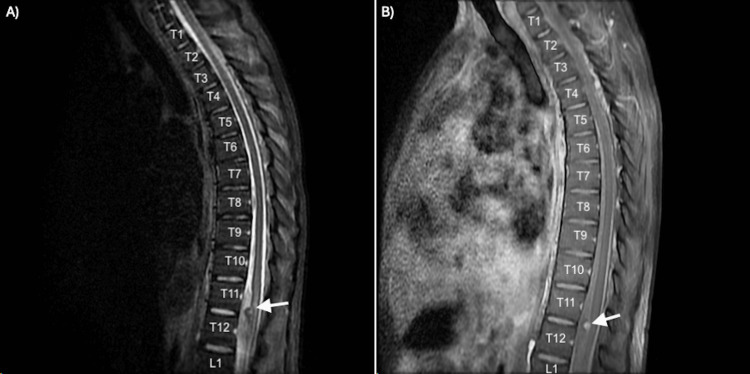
MRI of the thoracic spine with STIR sequence (A) displaying edema at the conus medullaris level adjacent to the site of the aneurysm and post-contrast T1-weighted sequence (B) showing enhancement of the aneurysm (aneurysm noted with an arrow). MRI, magnetic resonance imaging; STIR, short T1-inversion recovery
